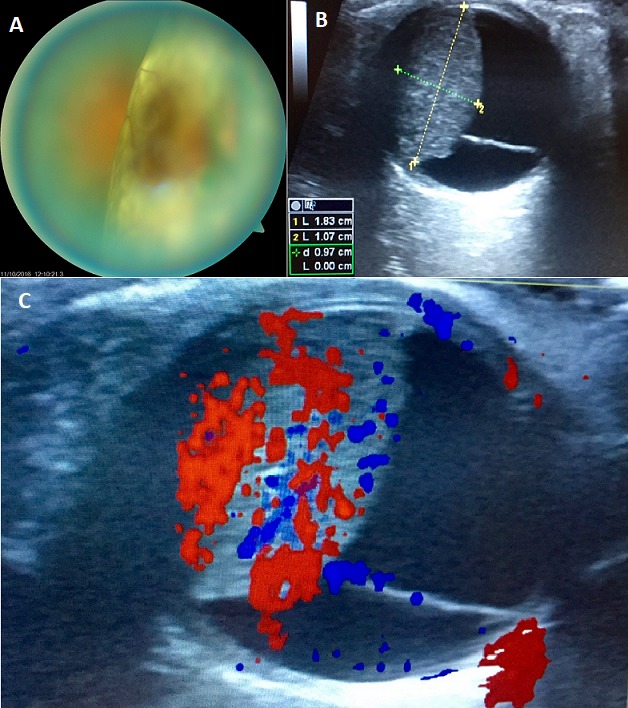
A) masse sous rétinienne occasionnant un décollement séreux; B) image échographique de la tumeur intraoculaire; C) vascularisation au Doppler de la tumeur intraoculaire
